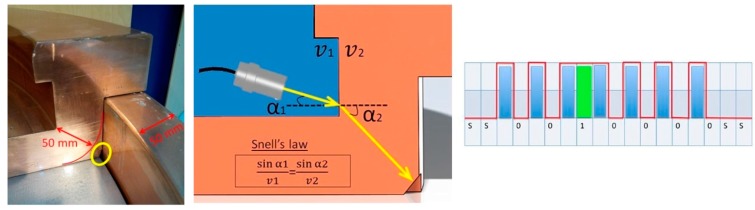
The ultrasonic investigation of chamfers, artificially machined on canisters’ lid to create a unique identification fingerprint.
